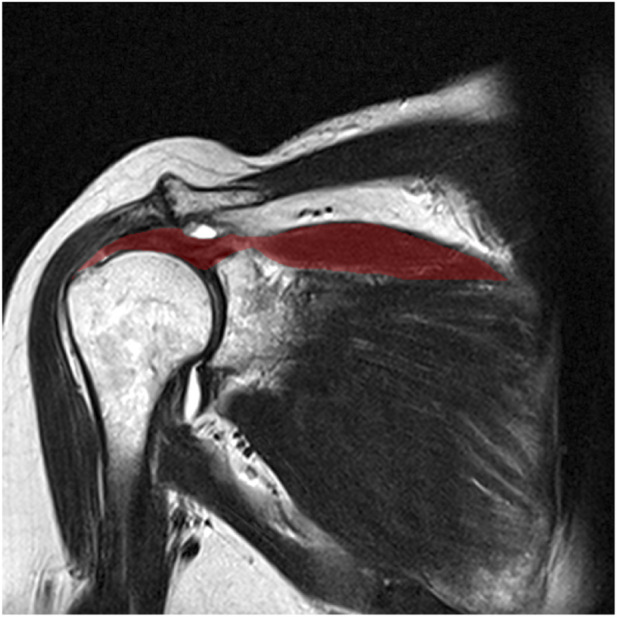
Manual segmentation of the supraspinatus muscle (shown in red) on a T2-coronal MRI slice using ITK-SNAP.
